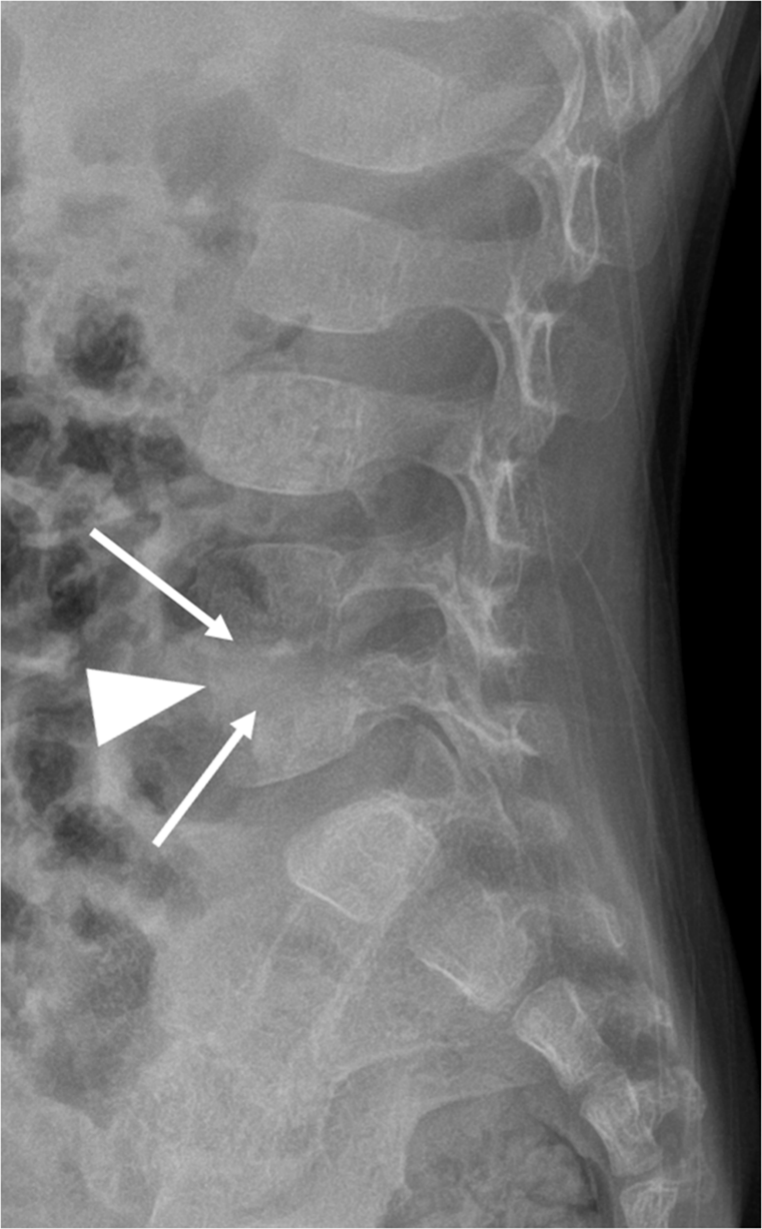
Lateral spinal radiograph of a 21-month-old girl with spondylodiscitis, obtained the same day as the ultrasound (Fig. 1), shows a narrowed space between the fourth and fifth lumbar vertebral bodies (*arrowhead*) with indistinct endplates (*arrows*)
